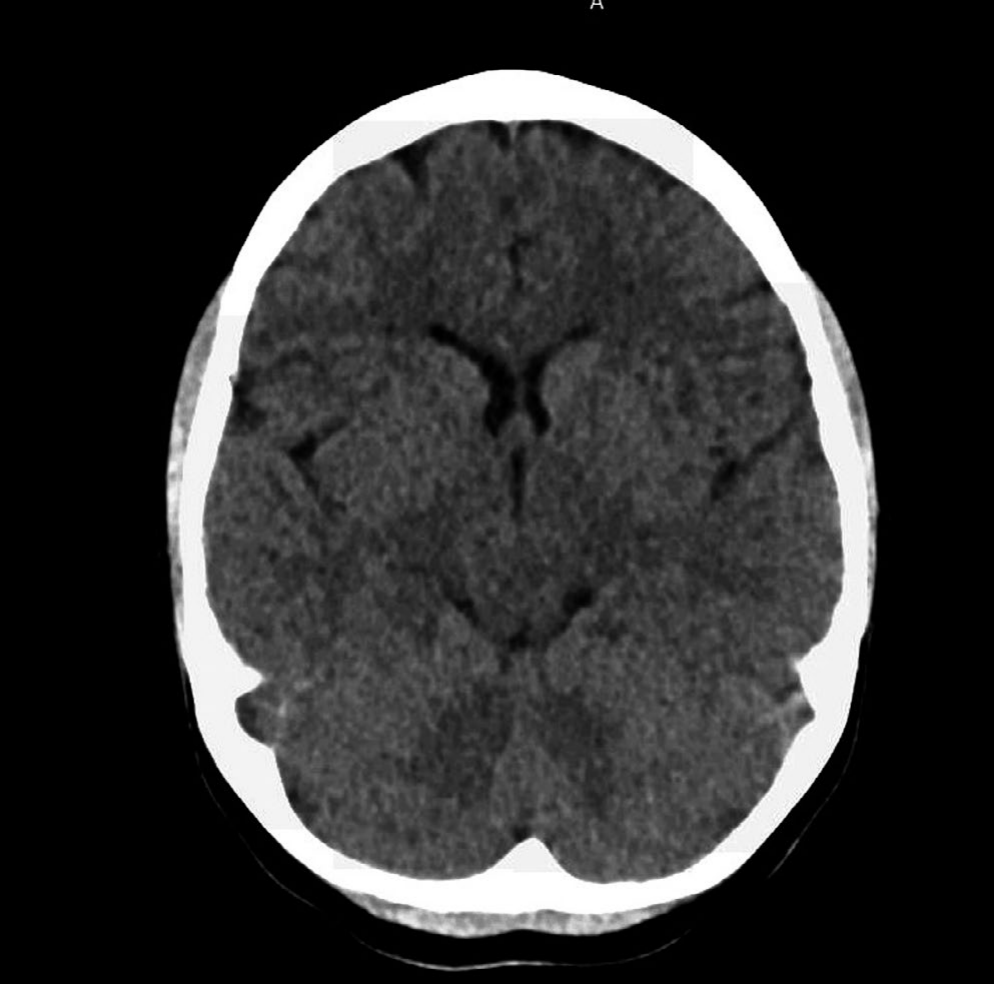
CT head without contrast performed at the time of presentation
